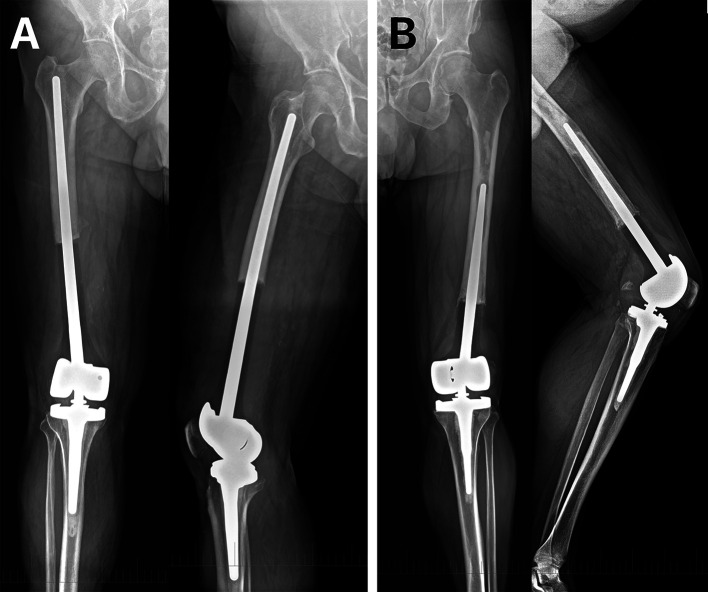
Representative radiographs of cemented custom-made distal femoral megaprosthetic reconstruction after tumor resection. (A) Patient following resection of a conventional grade 2 chondrosarcoma with a 20 cm distal femoral defect reconstructed using a cemented custom-made distal femoral replacement (stem diameter 14 mm, fixation length 21 cm). (B) Patient with malignant epithelioid hemangioendothelioma treated with distal femoral resection of 14 cm and reconstruction using a cemented custom-made megaprosthesis (stem diameter 16 mm, fixation length 17 cm), demonstrating aseptic loosening 6 years after implantation.
